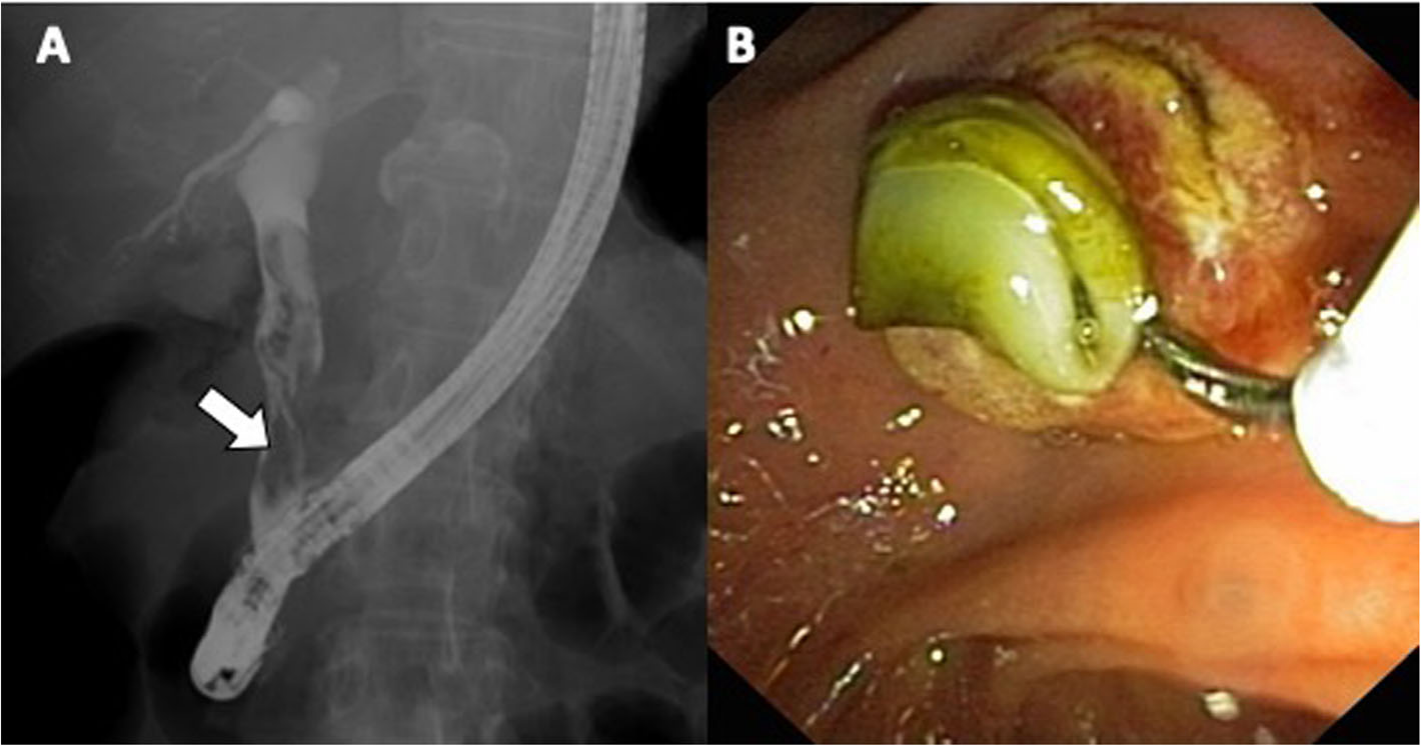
A 65-year-old woman with cysto-biliary rupture. a Radiographic image performed during ERCP procedure shows multiple filling defects into the common bile duct (arrow), representing hydatid membranes. b Endoscopic image of the same patient shows hydatid membranes extraction
